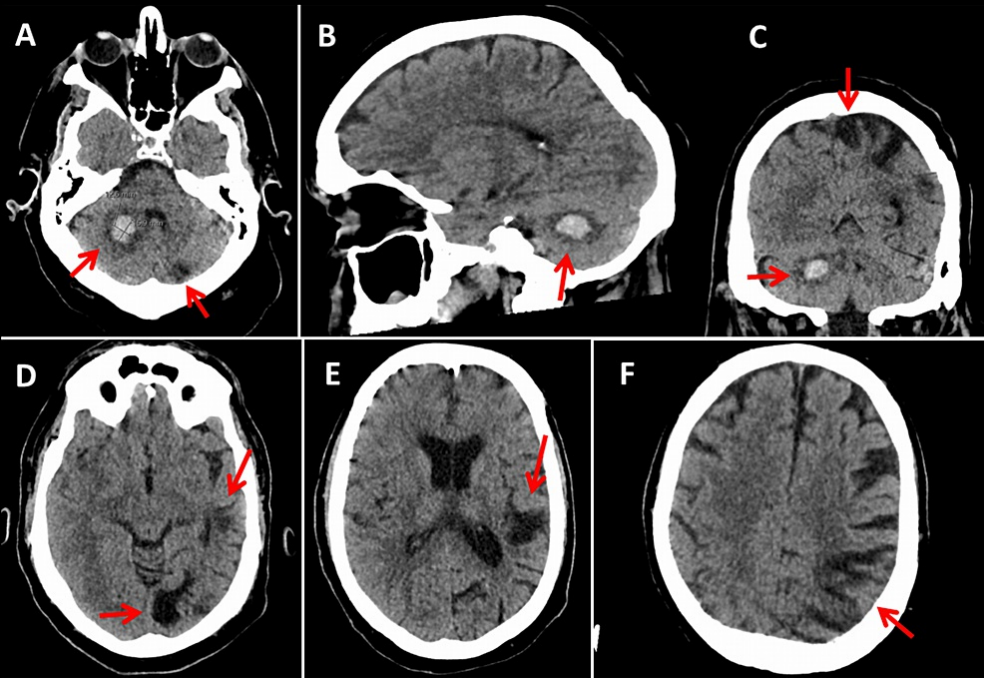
Cerebral CT showing an ischemic stroke with secondary bleeding in the right cerebellar hemisphere (A-C) (red arrows). In addition, old ischemic lesions can be seen in the area of the left posterior cerebral artery (D) and the left middle cerebral artery (D and F) (red arrows). CT: computed tomography
